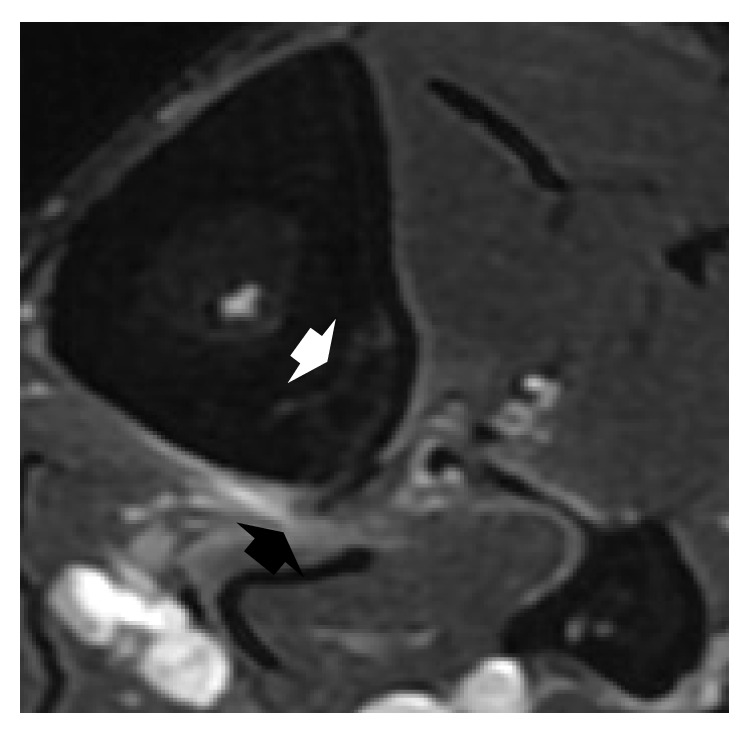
T2-weighted cross-sectional MR fat saturation sequence. The periosteum of the lateral margin of the tibia shows a hyperintense signal (*black arrow*) and hyperintense zones are visible in the lateral portion of the tibial diaphysis (*white arrow*).
